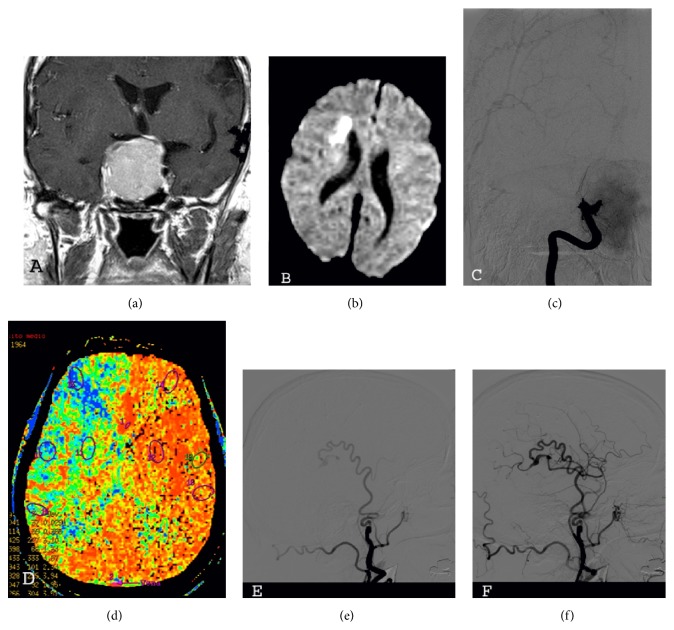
(a) Magnetic resonance showing the pituitary adenoma. (b) Magnetic resonance showing the ischemic lesion on the right side. (c) Digital subtraction angiography (DSA) showing the occlusion of the internal carotid artery at the level of the cavernous sinus on the right side. (d) Perfusion weighted CT scan: prolonged mean transit time on the right hemisphere. ((e) and (f)) Postoperative DSA (lateral view) showing the functioning of the bypass, early and late arterial phase.
